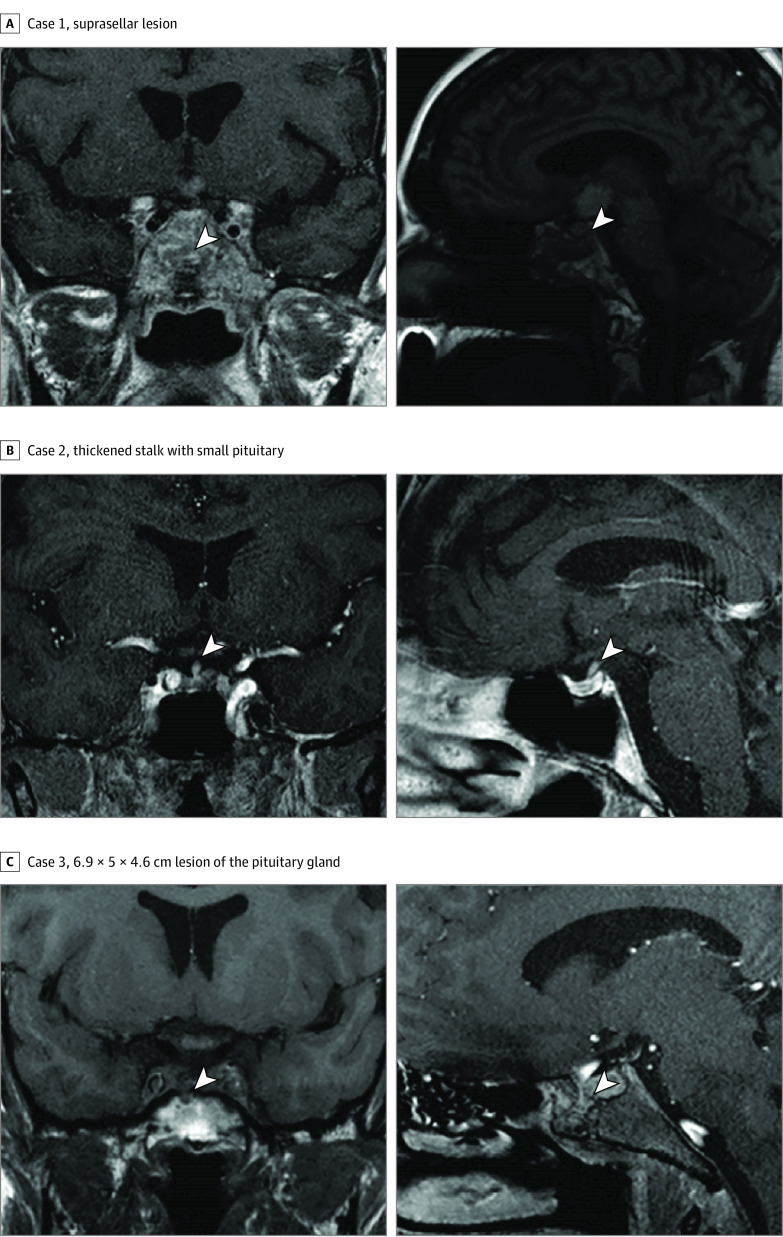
T1-Weighted Magnetic Resonance Imaging Scans of 3 Patients With Central Hypothyroidism
